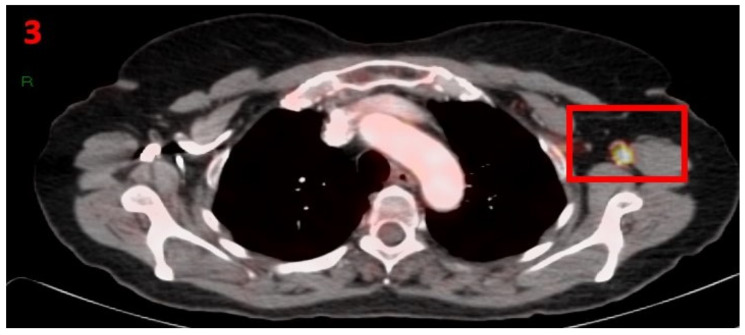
[18F]FDG PET-CT showing hypermetabolic uptake in the 3rd patient with lymphadenopathy in the left axillary region (red square).
